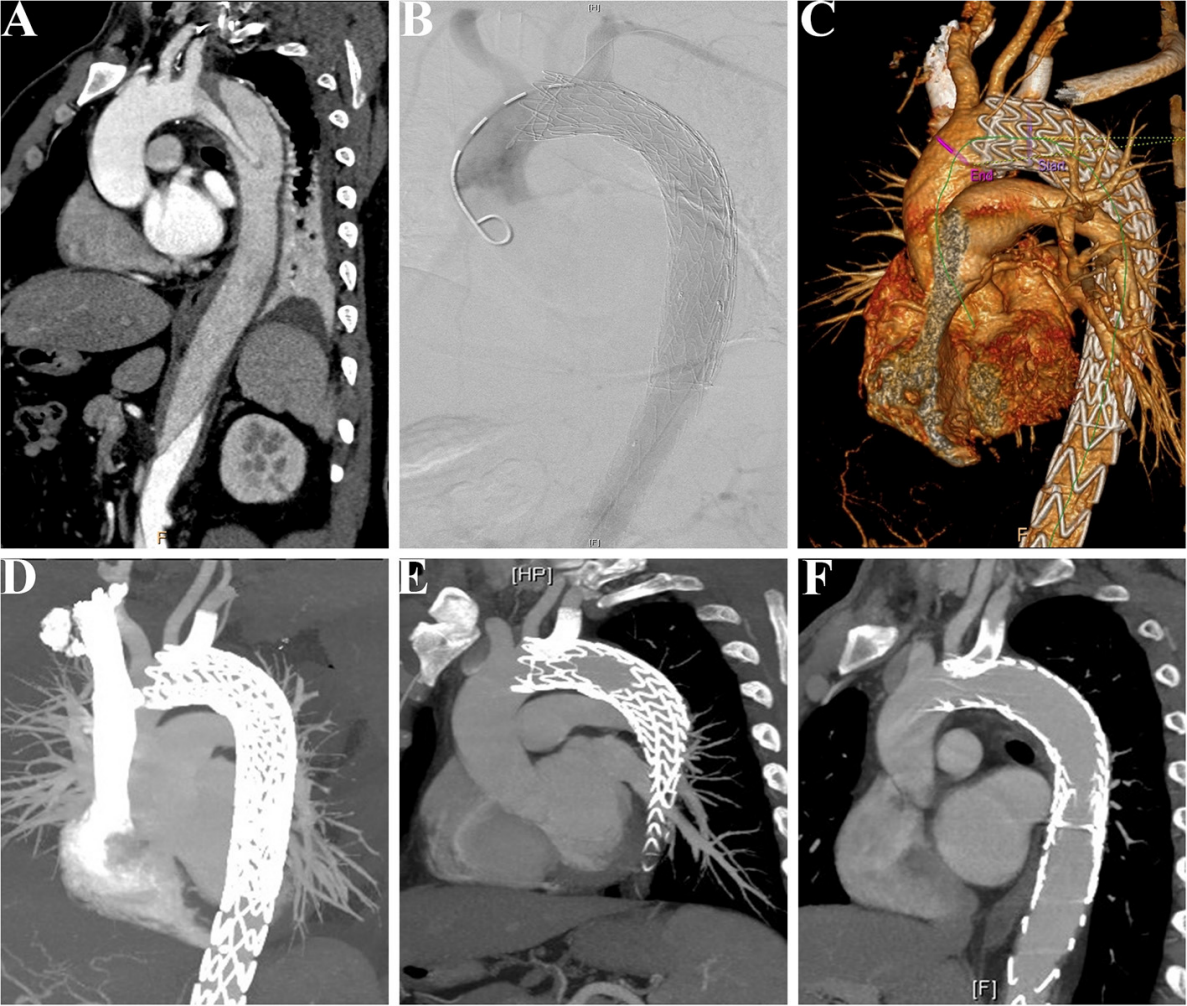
Patency of the LSCA was followed-up in a patient receiving the MCPF technique. (A) Preoperative CTA showed that a patient suffered from thoracic aortic dissection, which involved the LSCA. (B) Intraoperative aortogram demonstrated that the LSCA was revascularized and false lumen was excluded. (C) Before discharge, CTA was conducted to confirm the patency of the LSCA and exclusion of false lumen. (D) CTA at follow-up of 6 months. (E) CTA at follow-up of 12 months. (F) CTA at follow-up of 24 months. LSCA, left subclavian artery; MCPF, mini-cross prefenestration; CTA, CT angiography.
